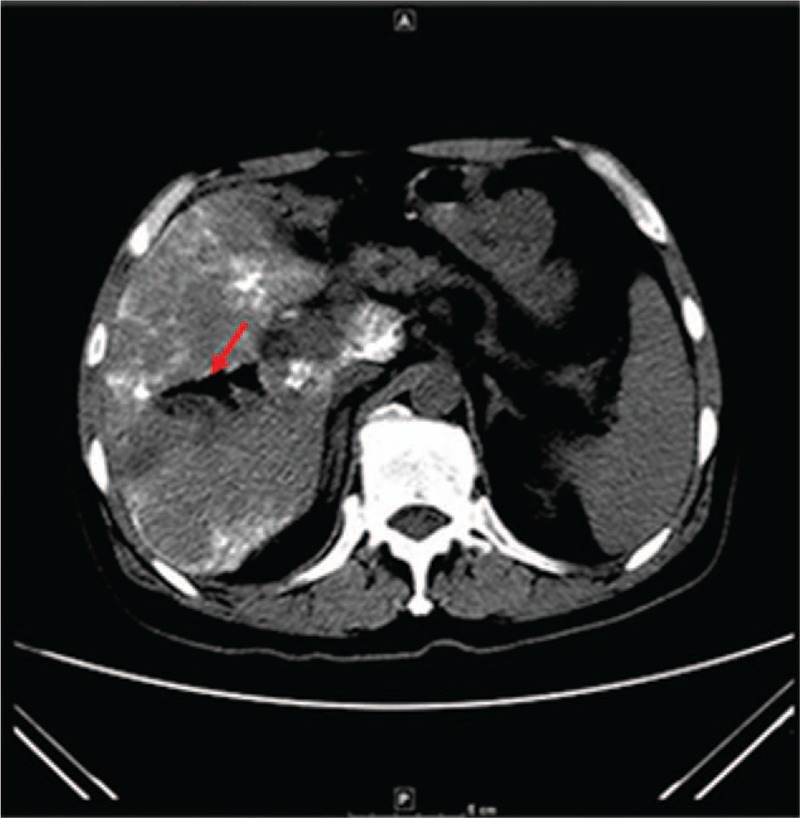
Abdominal CT scan, on January 3rd, 2018. Note: The red arrow represents accumulated gas in the liver.
